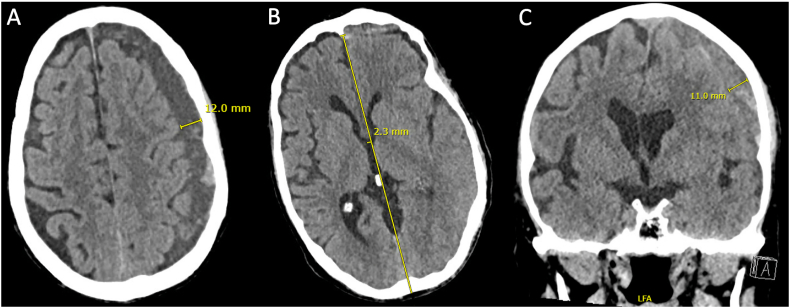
Initial Head CT, with left convexity SDH. Caption: Initial head CT with 11–12 mm left holohemispheric, multi-loculated SDH, with axial slices through the level of the corona radiata (Panel A) and coronal slice through the foramen of Monro (Panel C), with 2.3 mm of rightward midline shift (Panel B). CT = computed tomography; SDH = subdural hematoma.
